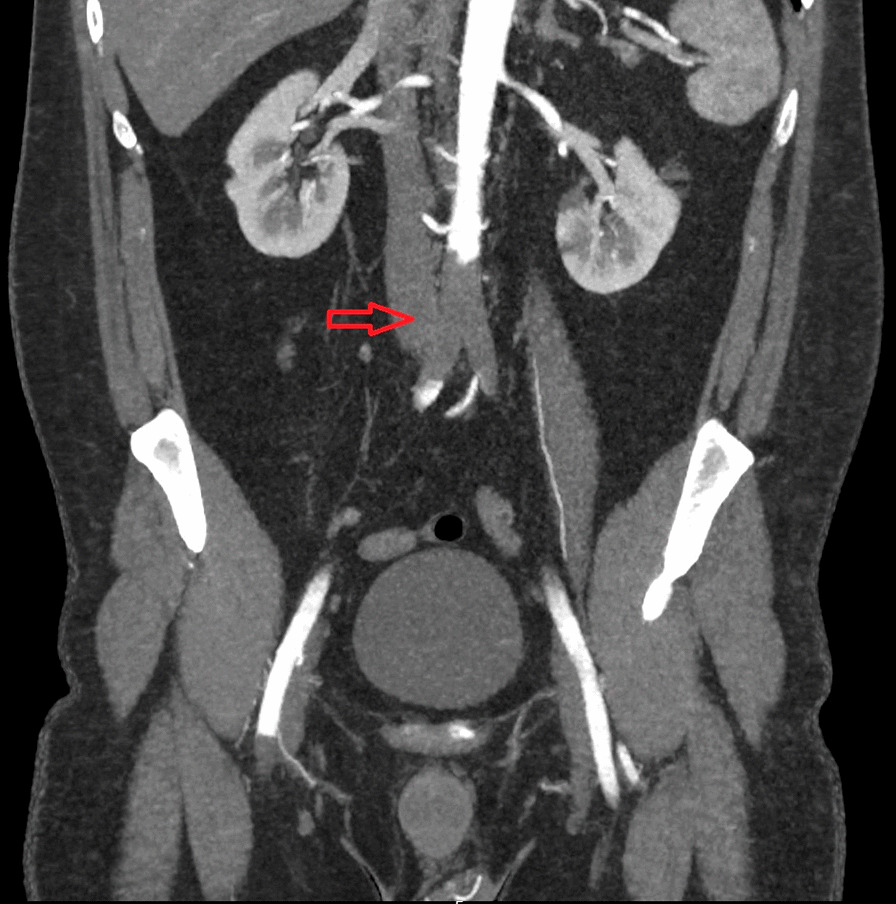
Thoracoabdominal computed tomography in coronal view during visit in the emergency department showing a massive thrombus in the aortoiliac region
